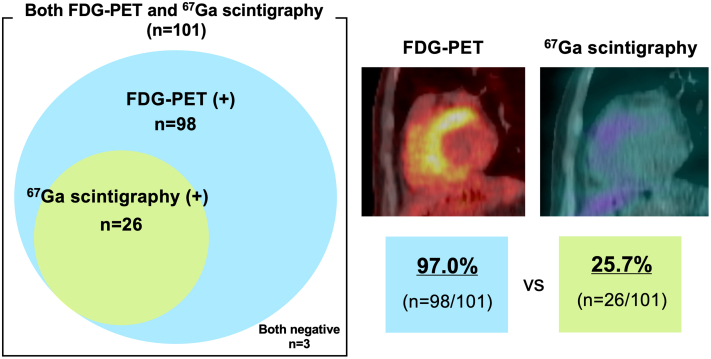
Distributions of Uptake in FDG-PET and ^67^Ga Scintigraphy FDG and ^67^Ga uptake were shown in 17 segments of the myocardium. Abbreviations as in Figure 1.
